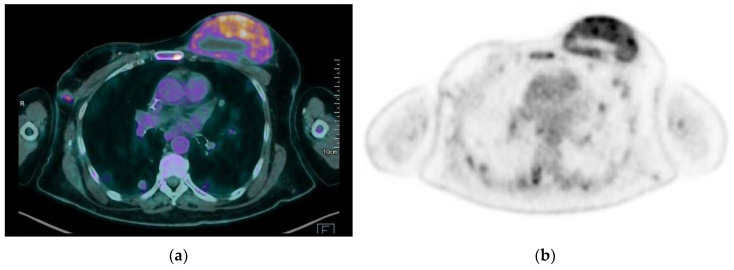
A 65-year-old patient with a history of PBA treated with right mastectomy, right axillary lymph node dissection, and radiotherapy of the right axilla. A 2-year follow-up with ^18^F FDG PET-CT scan revealed metastatic disease: (a,b) hypermetabolic lesions are observed in breasts, lungs, and bones.
